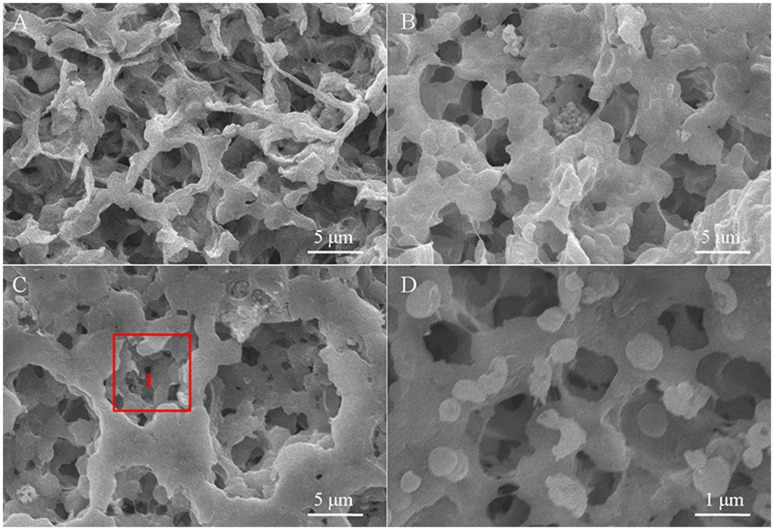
SEM images of the different CPE membranes (A, CPE-0; B, CPE-CT; C, CPE-LCT) and the partial enlarged SEM image of area 1 (D).
